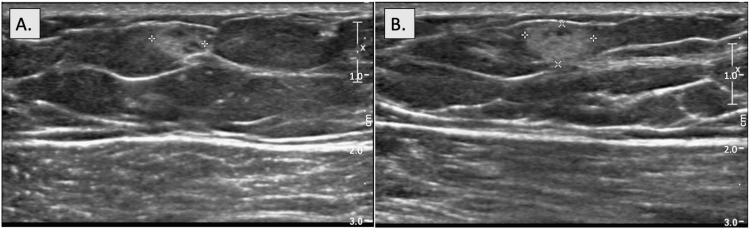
Sonography of left breast hemangioma Sonography performed in the left breast using radial (A) and anti-radial (B) approaches demonstrates an oval, solid mass with parallel orientation and indistinct margins measuring 9x6x7 mm at the 11 o’clock position, located 7 cm from the nipple. Internal echotexture is hyperechoic.
